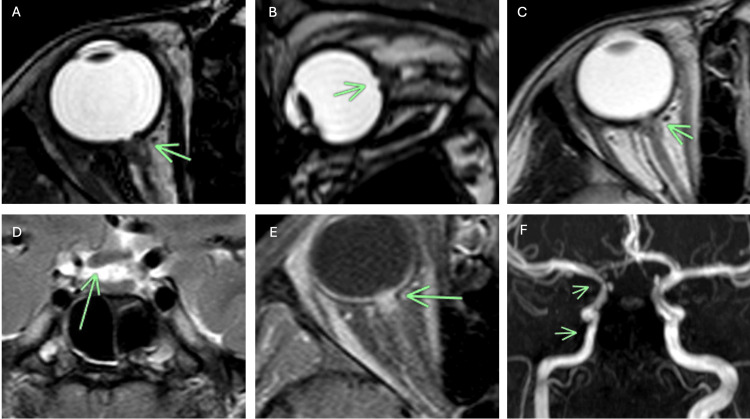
Imaging findings in a patient with right orbit morning glory disc anomaly (MGDA) Balanced fast field echo (BFFE) MR sequence in the axial plane (A) and sagittal plane (B) through the right orbit shows a funnel-shaped morphologic structure of the optic disc (arrow) with uplift of the adjacent retinal surface. This image also shows abnormal tissue, which is associated with the terminal of the intraorbital segment of the right optic nerve and subarachnoid space compression of this level (arrow). The vascular layer of uveosclerosis at the site of optic nerve entry is also intermittent. C) The axial T2-weighted image revealed focal effacement of the perioptic CSF space caused by abnormal thickening of the soft tissue at the end of the intraorbital segment of the right optic nerve(arrow). (D) The T2-weighted coronal image represents asymmetric thickening of the right optic chiasm (arrow). (E) The axial T1-post contrast image shows abnormal enhancement in the distal retrobulbar area of the right optic nerve, probably due to displaced choroidal tissue, which is accompanied by glial, fibrous, and pigment epithelial proliferation. (F) MRI angiography across the Circle of Willis showed severe vascular abnormalities of the right internal carotid artery system (ICA). The intracranial ICA right side segments, including the petrous, cavernous, and supraclinoid, appeared to be narrow. The right anterior cerebral artery (A1) was hypoplastic. The rest of the circle of Willis and the vertebrobasilar system looked normal. No evidence of abnormal vascular collateralization, indicating the presence of Moyamoya disease, was found at this point.
